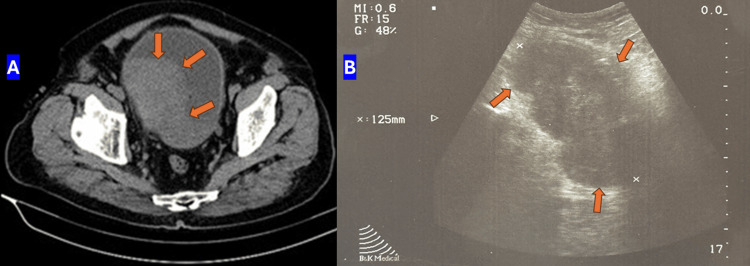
Tumor formation located in the bladder (orange arrows). A) Computed tomography of bladder; B) Ultrasound of bladder.
